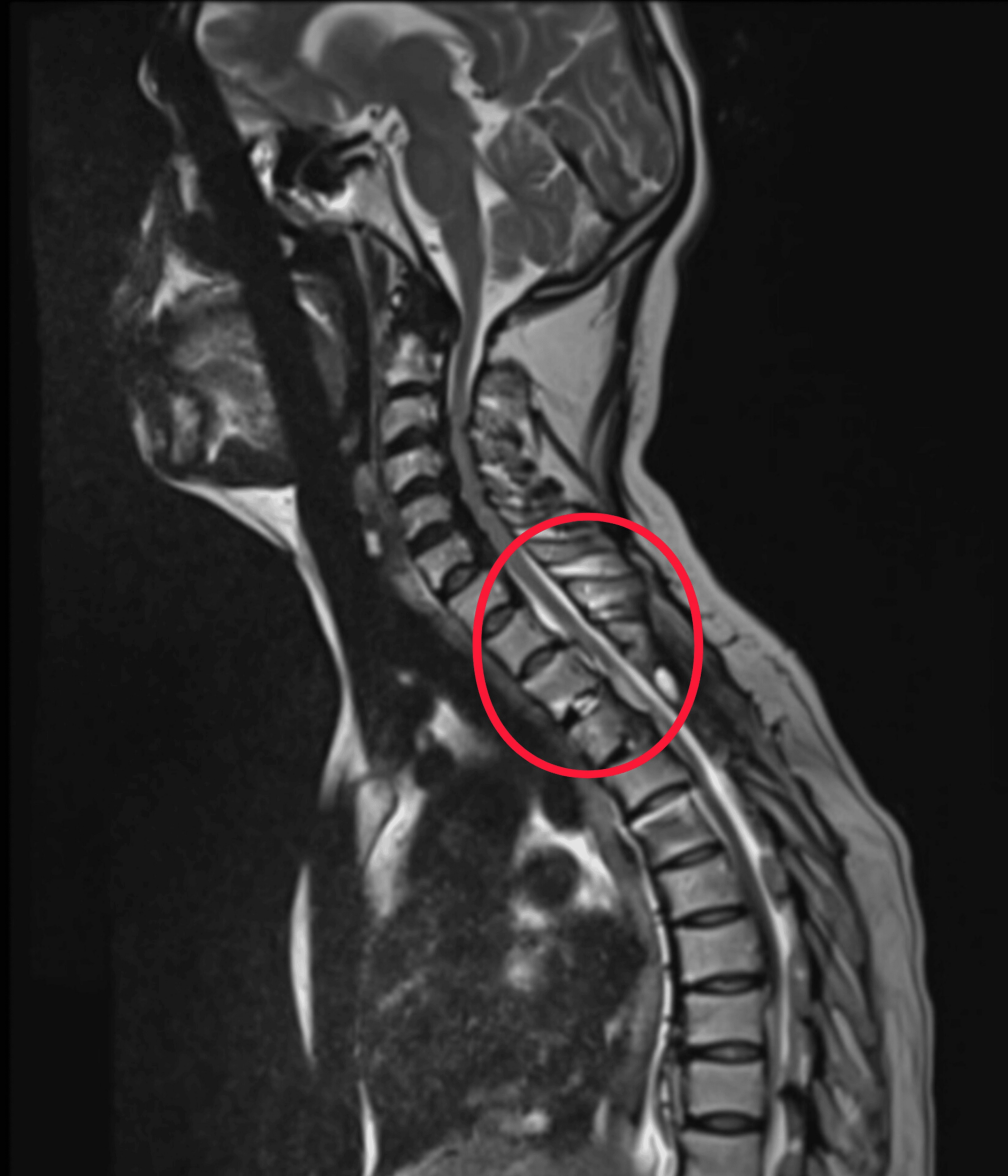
Post-operative magnetic resonance imaging (MRI) demonstrates restoration of CSF space and relieved spinal cord compression at the T3-T4 level (red circle). CSF: cerebrospinal fluid
